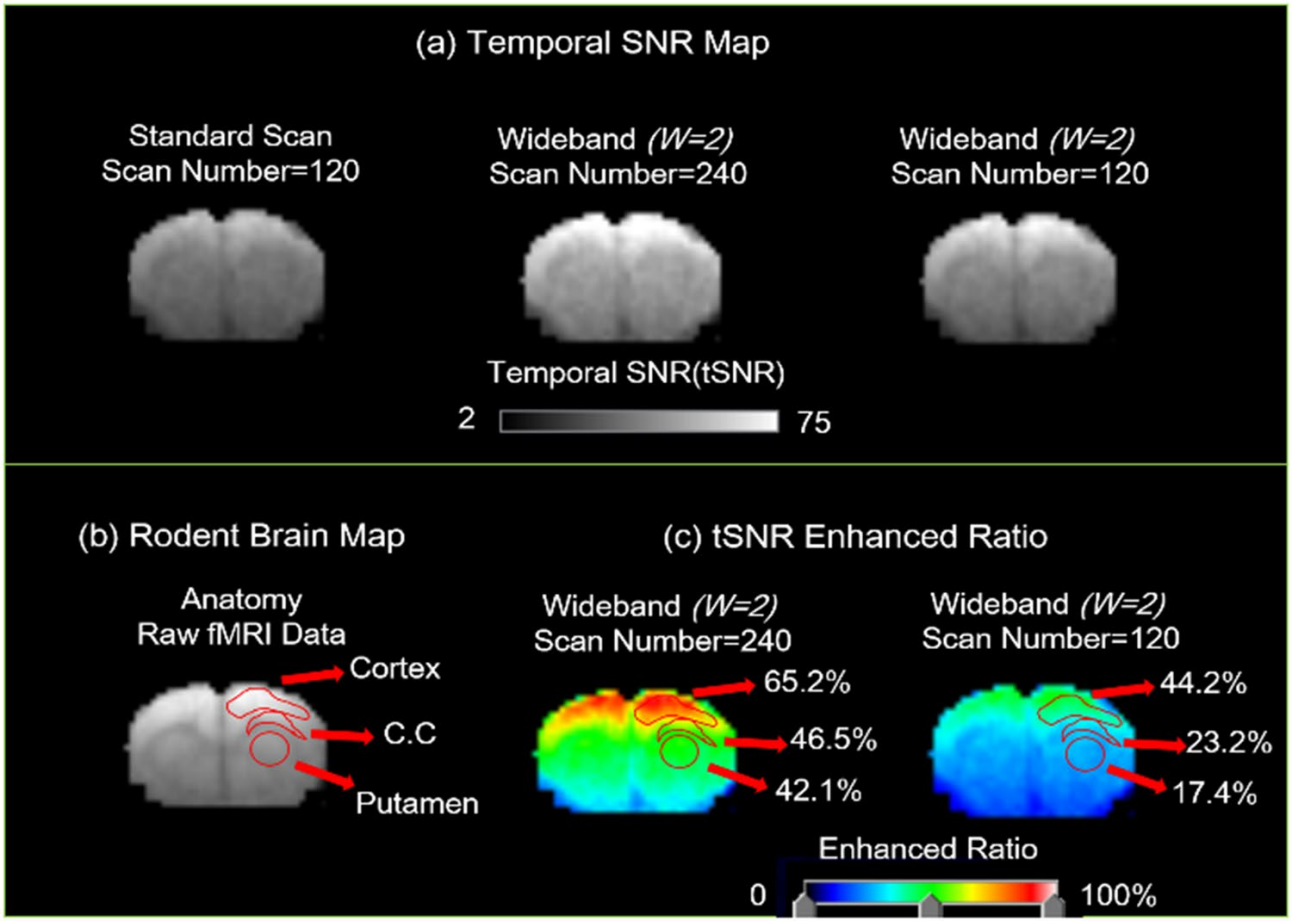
Temporal signal to noise ratio map. In the resting functional MRI experiment, the tSNR map was boosted by 65.2% to 70.9 ± 9.22 by doubling the scan number, and was elevated from 43.28 ± 9.65 to 62.41 ± 9.52 (44.2%) by shorten the sampling interval in the cortex area. In the corpus callosum and Putamen, by doubling the scan number in the cortex area, tSNR increased by 46.5% and 42.1%; by shortening the sampling interval, tSNR increased from 34.23 ± 11.28 and 32.12 ± 13.22 to 42.17 ± 9.31 (23.2%) and 37.71 ± 9.28 (17.4%).
